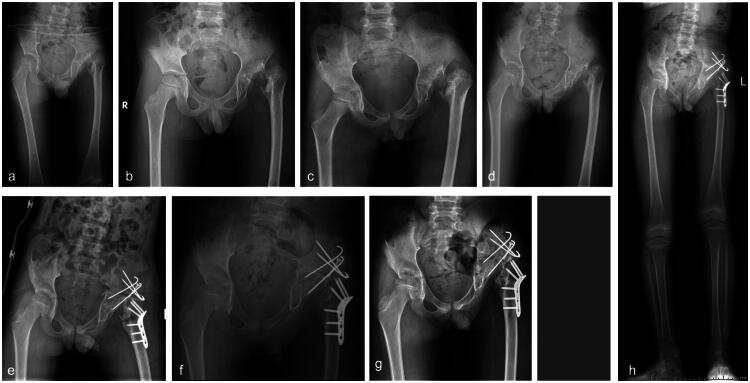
a–h The case 11, a child aged 9–10 years, developed left-sided SAH at less than 2 years of age, which caused a gait abnormality noticed when he was five years old. A pelvic X-ray revealed left hip dislocation with complete femoral neck resorption and residual femoral head (type IIB) (a). No improvement was observed during consecutive follow-ups (b–d), and he developed left hip pain. As a result, he underwent acetabular lateral Chiari osteotomy combined with proximal femoral valgus osteotomy (e–g). Recent follow-up showed osteotomy healing, hip stability and good hip function, but a residual LLD of 3 cm remains (h).
